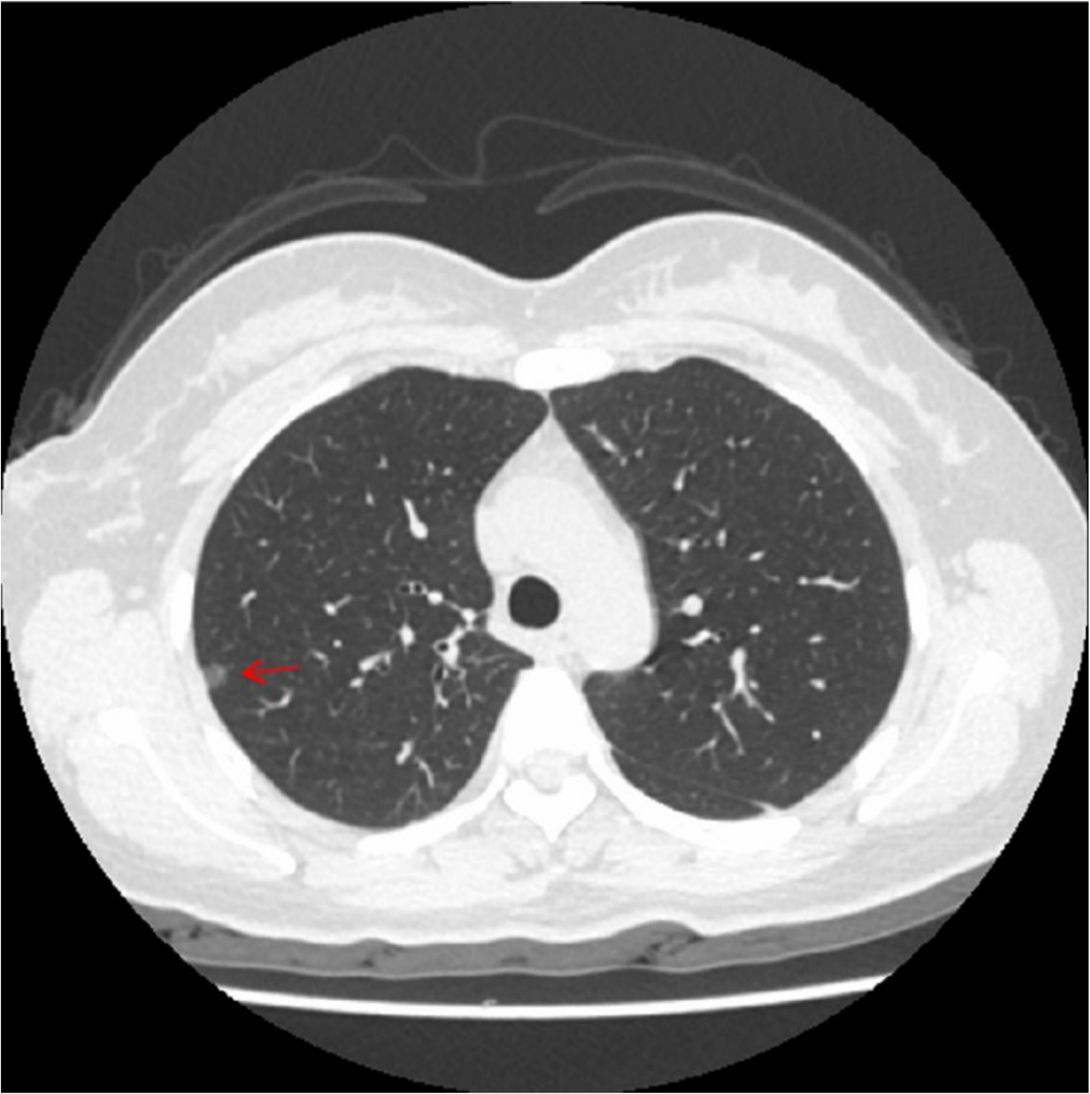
The preoperative computed tomography scanning. A 7-mm ground-glass nodule was identified at the posterior segment of the right upper lobe (arrow), the CT value was -400HU
